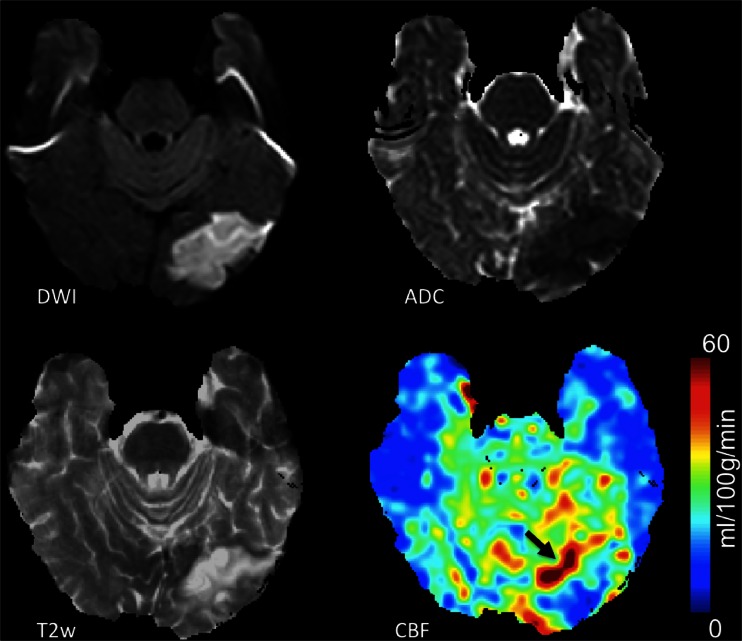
Acute ischaemia in the left occipital lobe, with diffusion restriction on the diffusion-weighted image (*DWI*) and apparent diffusion coefficient (*ADC*) map, and high signal intensity on the T2 weighted (*T2w*) images. The colour-coded cerebral blood flow (*CBF*) map shows hypoperfusion in the ischaemic region, with the *arrow* indicating the residual vascular signal in the arteries feeding the ischaemic tissue (arterial transfer artefact: *ATA*)
